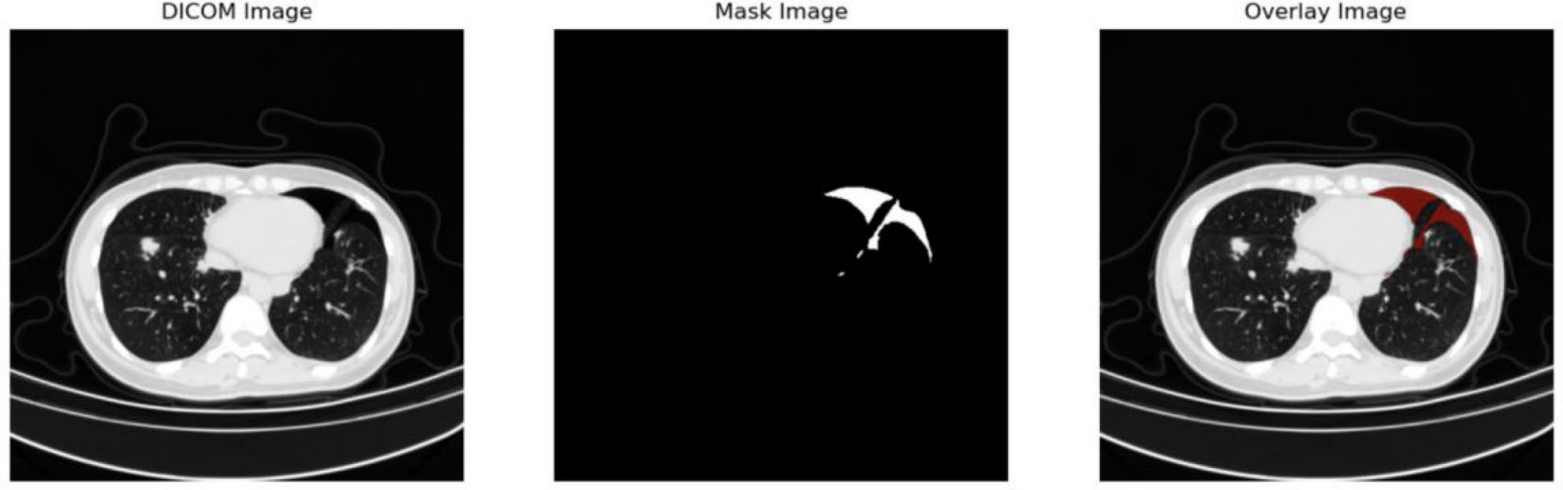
Examples of 3 different CT images (left), the masks (middle), and the CT images with masks (right).
